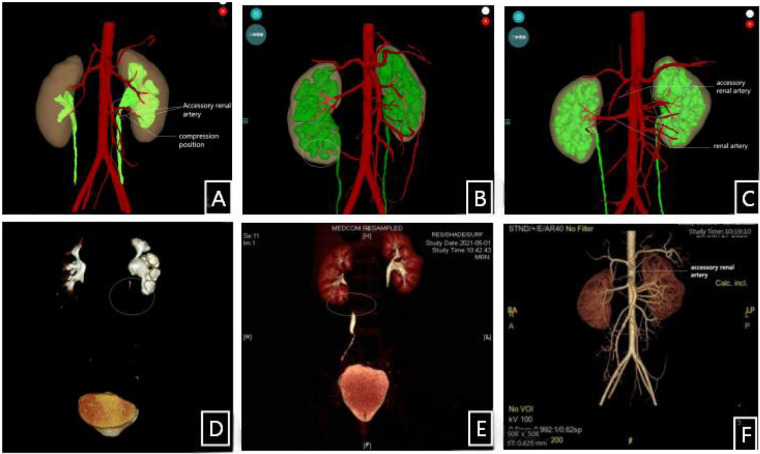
A comparison of CAS 3D reconstruction, CTU, and CTA images. (A–C) CAS 3D images clearly visualize the thin pediatric ARA. (D–F) Conventional CTU and CTA fail to clearly delineate the ARA.
